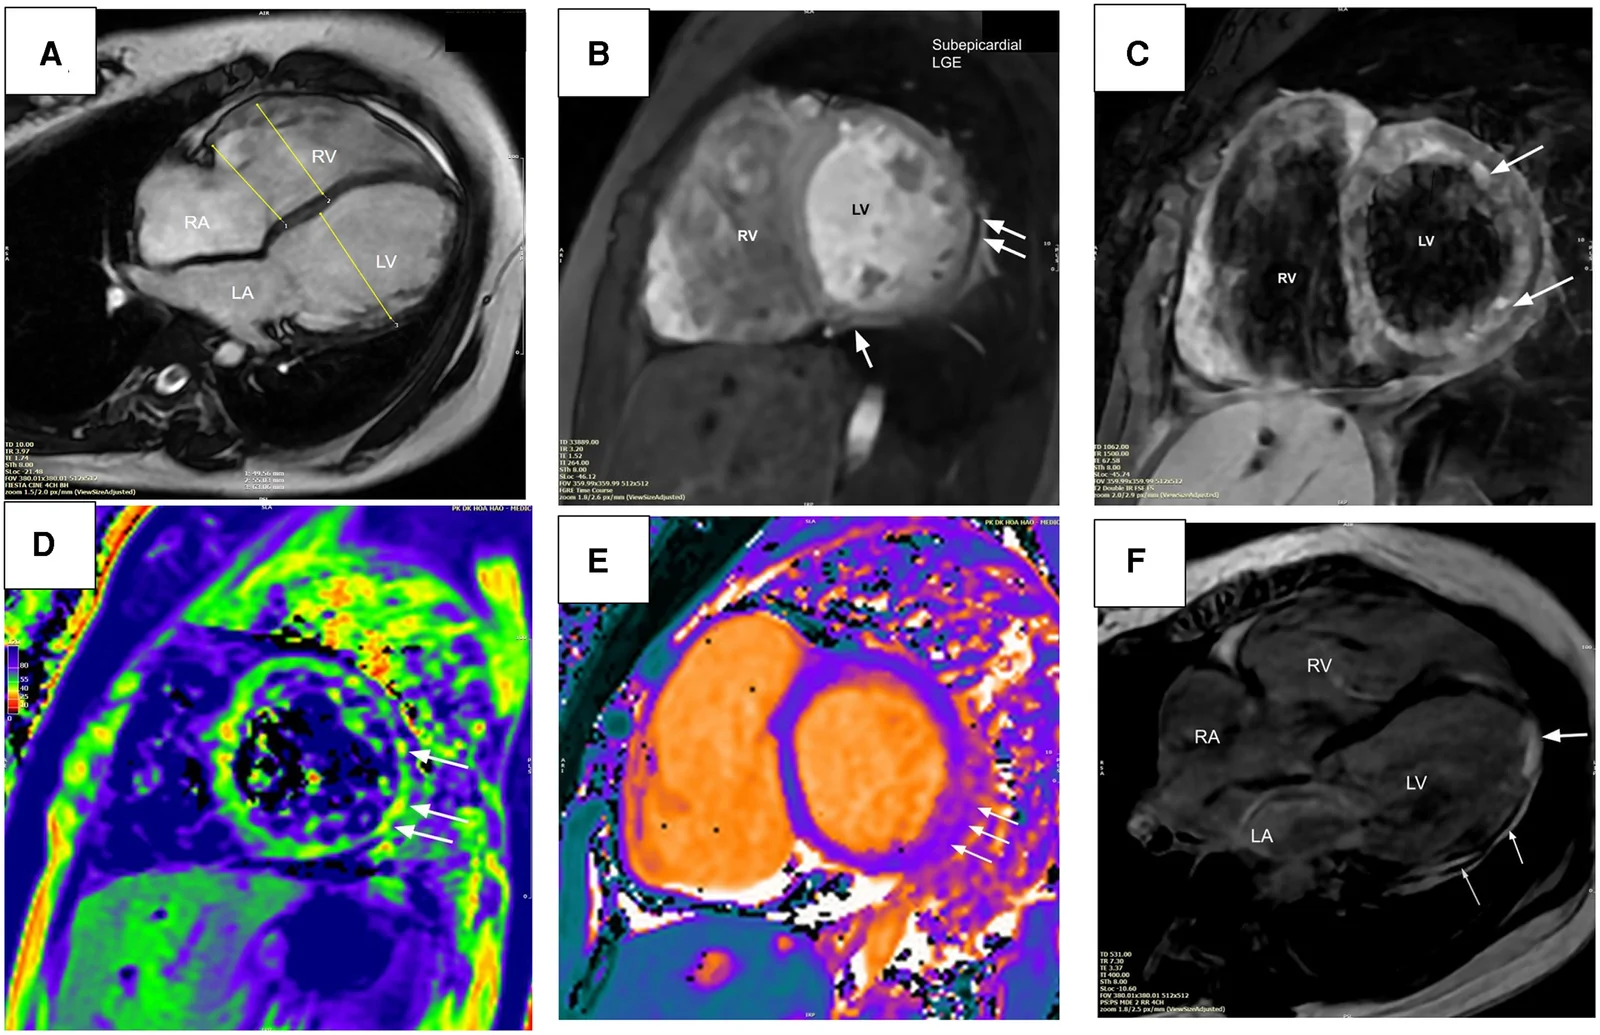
Cardiac magnetic resonance imaging. (*A*) Four-chamber cine view demonstrating biventricular dilation with severely reduced systolic function. (*B*) Late gadolinium enhancement short-axis view showing patchy subepicardial and mid-wall enhancement (arrows), predominantly involving the lateral wall and right ventricular insertion point. (*C*) T2-weighted double inversion recovery imaging demonstrating increased myocardial signal intensity (arrows), consistent with myocardial oedema. (*D*) T2 mapping demonstrating increased myocardial T2 values (arrows), predominantly involving the lateral and inferolateral walls. Elevated native T2 relaxation times (Native T2 = 68 ms) (*E*) Native T1 mapping showing elevated myocardial T1 values (arrows), consistent with diffuse fibrosis. Quantitative analysis demonstrated elevated native T1 (1107 ms) and extracellular volume (42%). (*F*) Long-axis late gadolinium enhancement view confirming subepicardial enhancement involving the lateral wall, supporting a non-ischaemic pattern of myocardial fibrosis.
